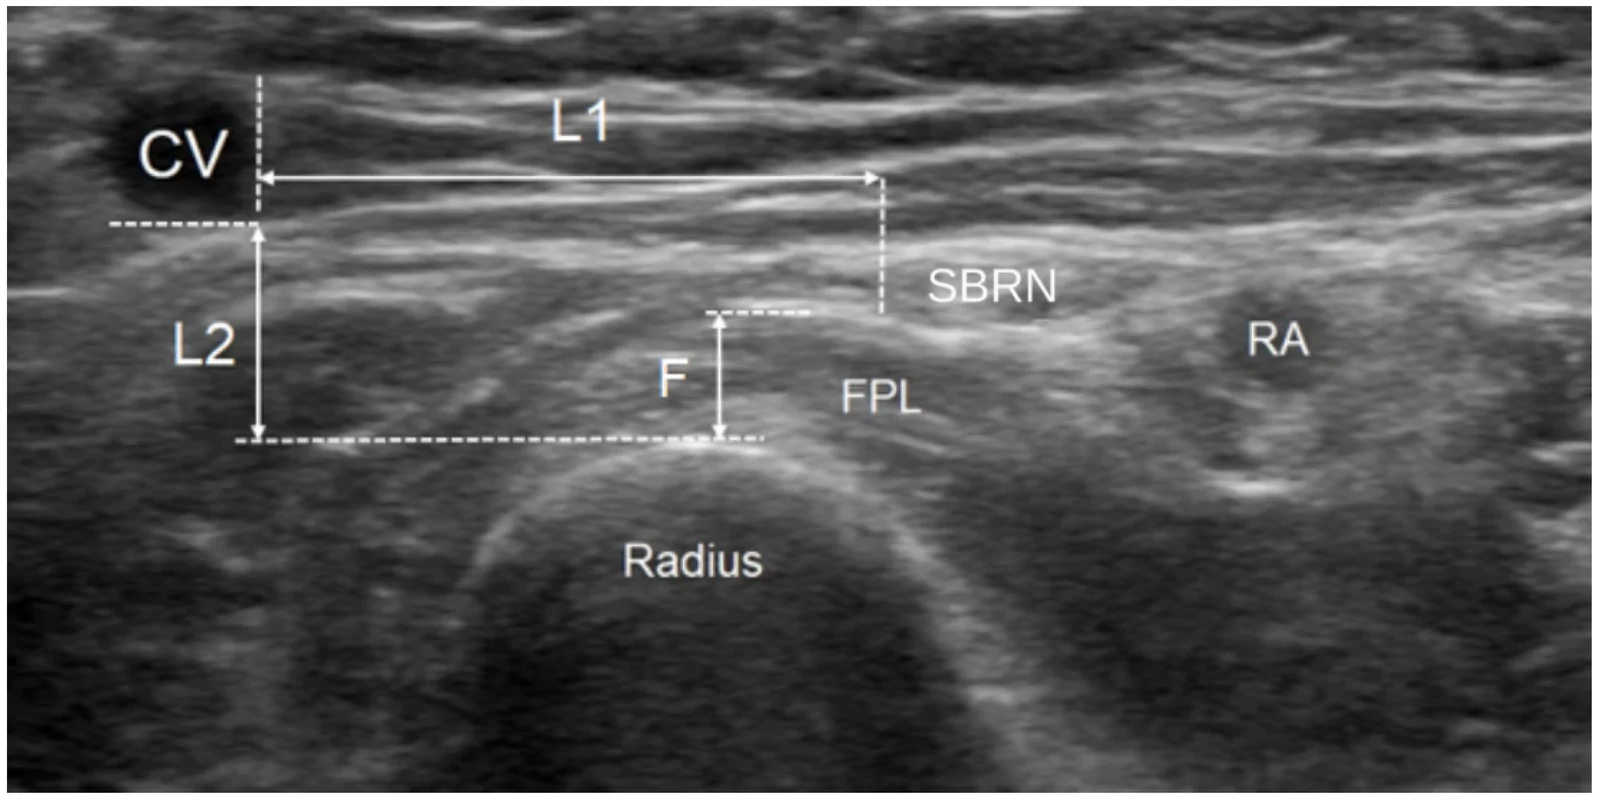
Transverse ultrasonographic view of the distal third of the forearm. Solid double-headed arrows indicate the measured distances; dashed lines mark the reference borders from which each measurement was taken. L_1_, distance between the medial wall of the CV and the lateral border of the SBRN; L_2_, distance between the inferior wall of the CV and the apex (i.e., the highest visible point) of the radial cortex; F, FPL exposure height. CV, cephalic vein; FPL, flexor pollicis longus; RA, radial artery; SBRN, superficial branch of the radial nerve.
